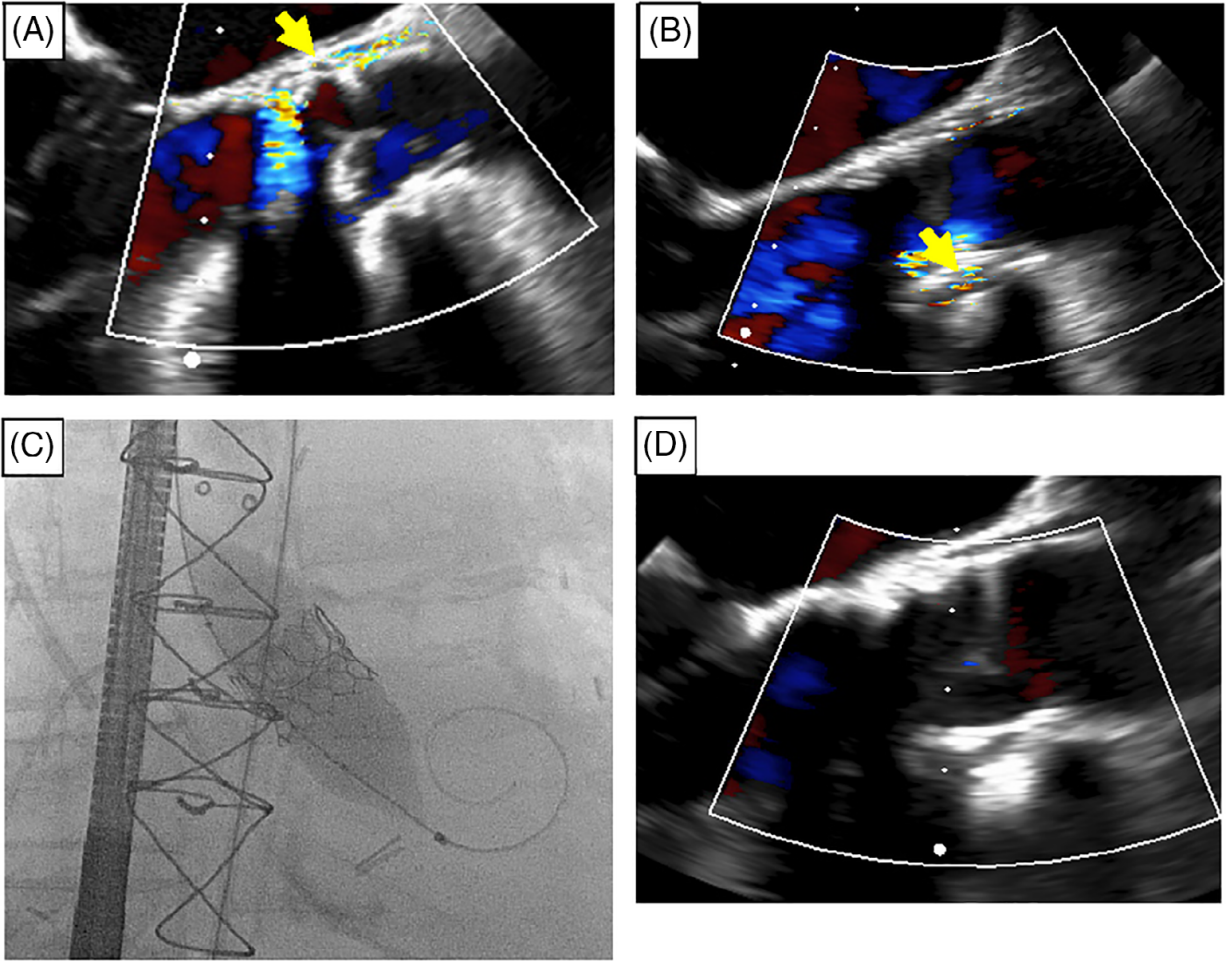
Intraoperative images of patient 2. A, TEE image of first PVR jet prior to procedure (yellow arrow; Supplemental Video 4). B, TEE image of second PVR prior to procedure (yellow arrow; Supplemental Video 5). C, Cine angiography of balloon post‐dilation (Supplemental Video 6). D, TEE image after completion of the procedure (Supplemental Video 7). PVR, paravalvular regurgitation; TEE, transesophageal echocardiogram; ViV, valve‐in‐valve
